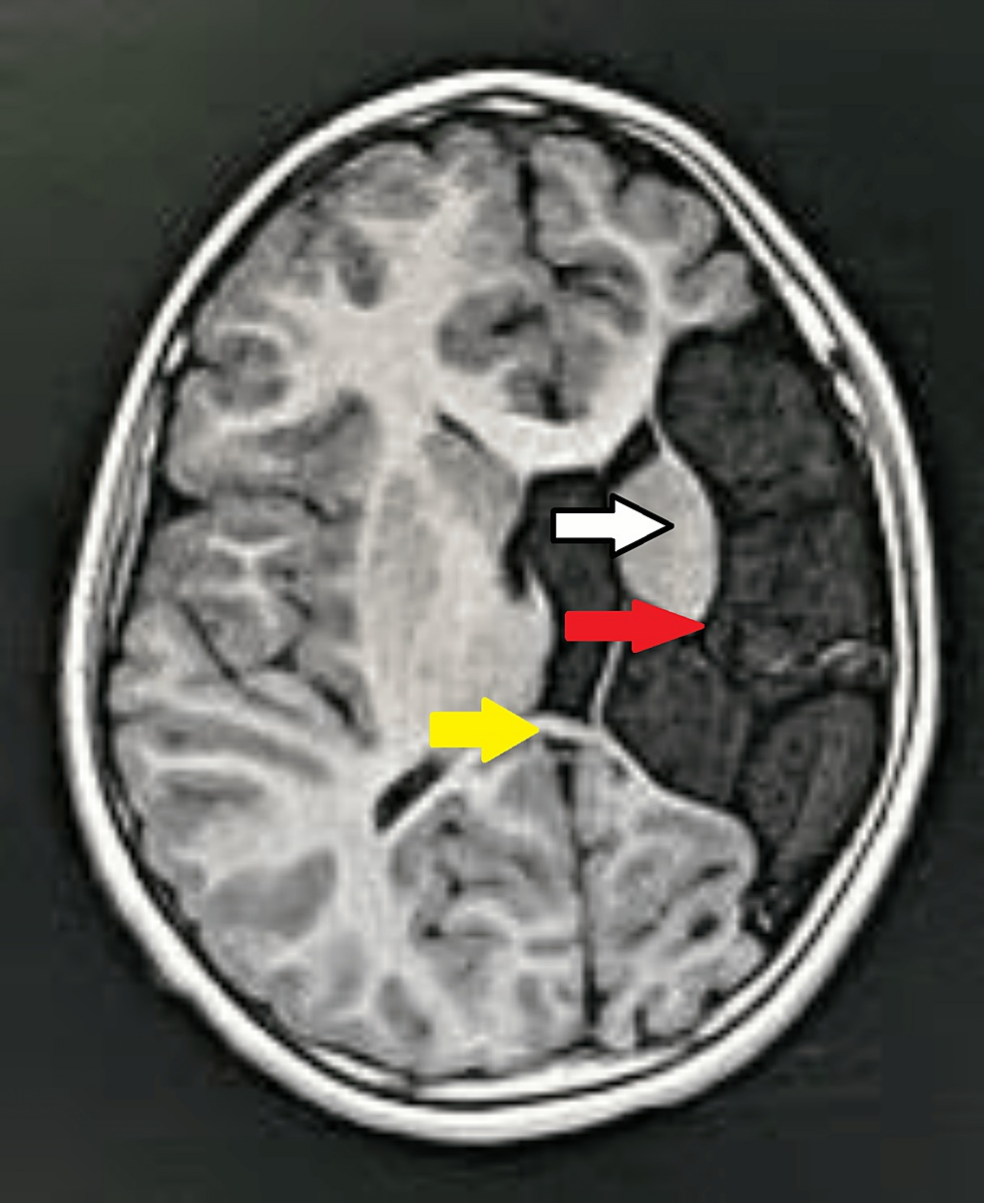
MRI brain axial T1WI Altered signal intensity is noted at the frontoparietal lobe on the left side with the loss of gray-white matter junction, encephalomalacia, and gliotic changes with dilatation of anterior horn and body of ipsilateral lateral ventricle appearing hypointense on T1WI suggestive of significant left-sided partial cerebral hemiatrophy. Atrophy of the splenium of the corpus callosum, lentiform nucleus, basal ganglia, and internal capsule on the left side is noted. Red arrow: altered signal intensity with encephalomalacia, and gliotic changes at the left frontoparietal lobe. White arrow: atrophic left gangliocapsular region. Yellow arrow: atrophic splenium MRI: magnetic resonance imaging; T1WI: T1 weighted imaging
